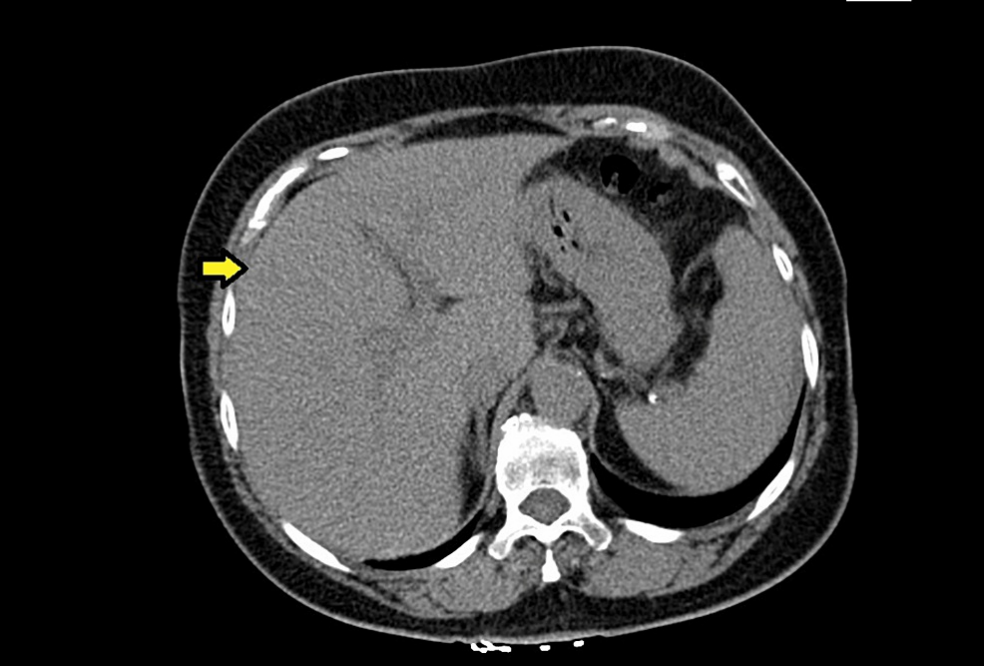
Repeated CT scan showing a complete resolution of previously seen ascites on the PET scan (yellow arrow). CT: computed tomography, PET: positron emission tomography
